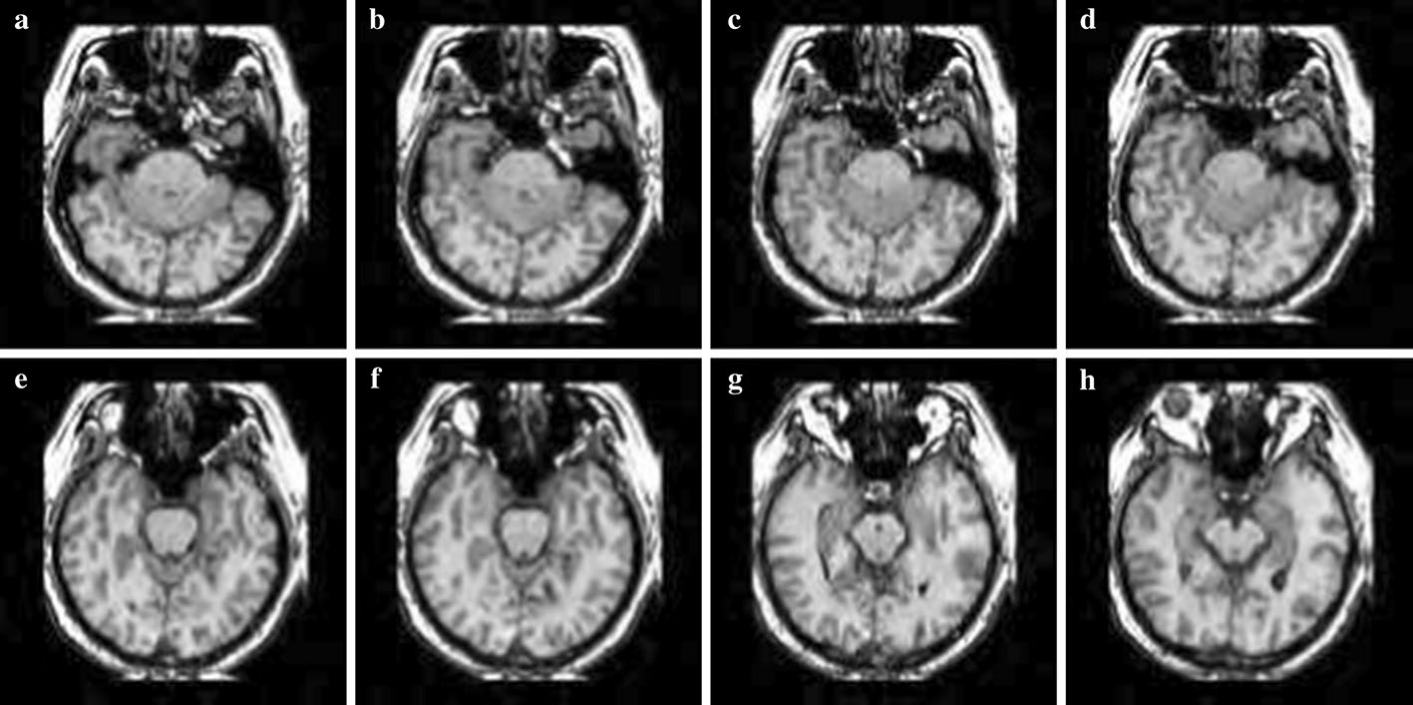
Compressed MRI image slices (a–h) by Jyotheswar and Mahapatra (2007) at a BR = 1.0 bpp
